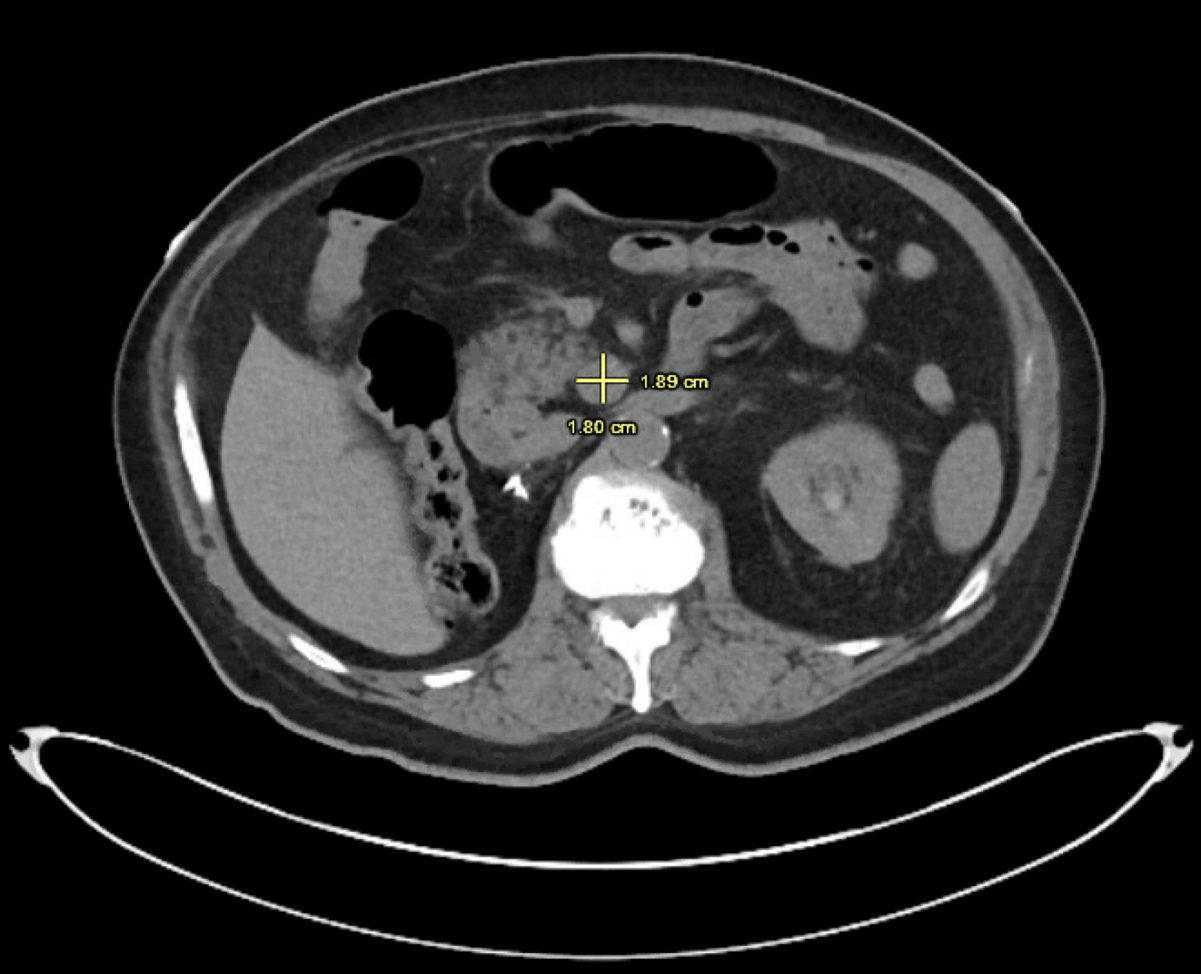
CTAP showing enlarged LN measuring 1.8 cm x 1.9 cm at the head of pancreas (dimensions marked in yellow). CTAP, computed tomography abdomen and pelvis; LN, lymph node
